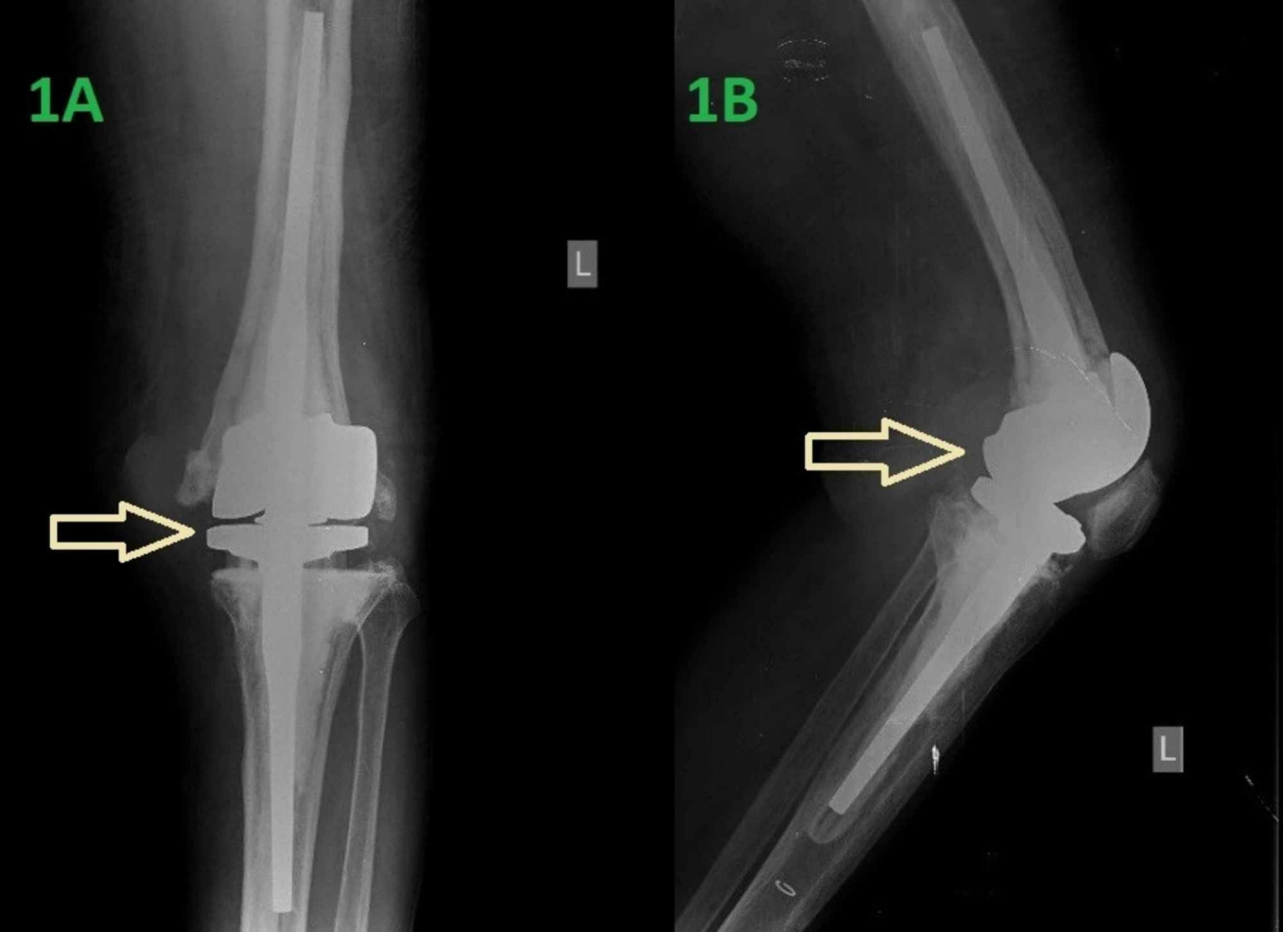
Initial X-rays of the patient's knee revealing the revision prosthesis (A): anteroposterior view (B): lateral view
